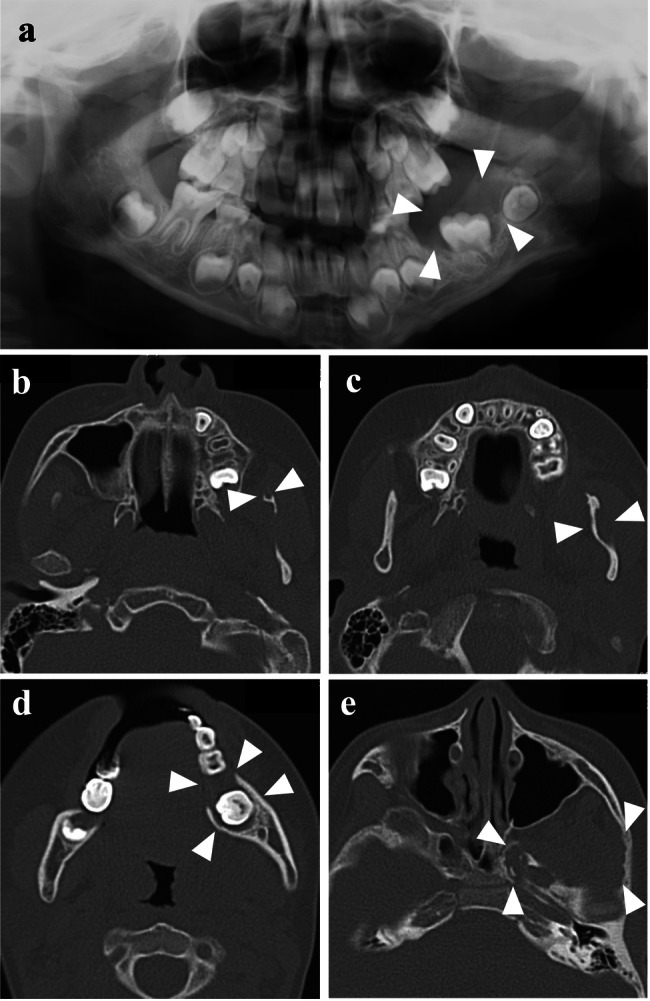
Panoramic radiography and CT imaging of the mandible. a Panoramic radiography at the initial examination (age 7). b–e CT imaging revealed multiple neurofibromas at age 9 in the left mandibular coronoid process (b), left mandibular ramus (c), left mandibular body (d), and adipose tissue of the left masticator space (e). Arrowheads indicate neurofibromas.
